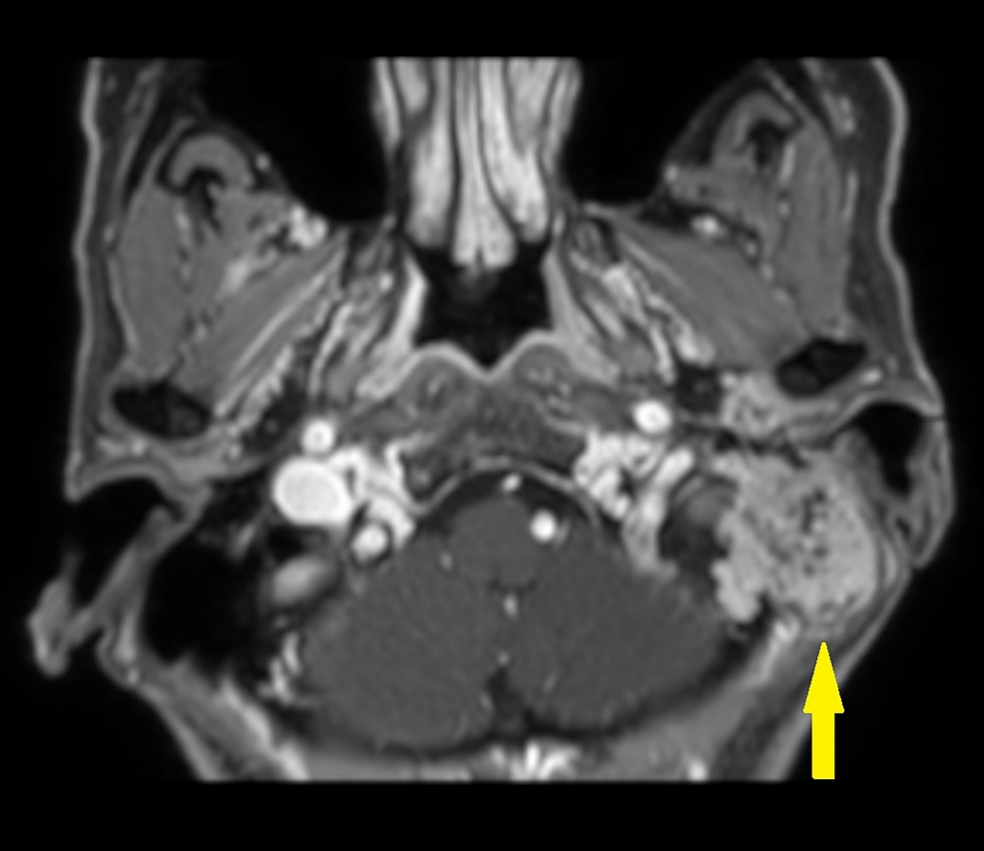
MRI at first visit Gadolinium-enhanced fat-saturated T1-weighted image Yellow arrow: tumor located in the left parotid gland
